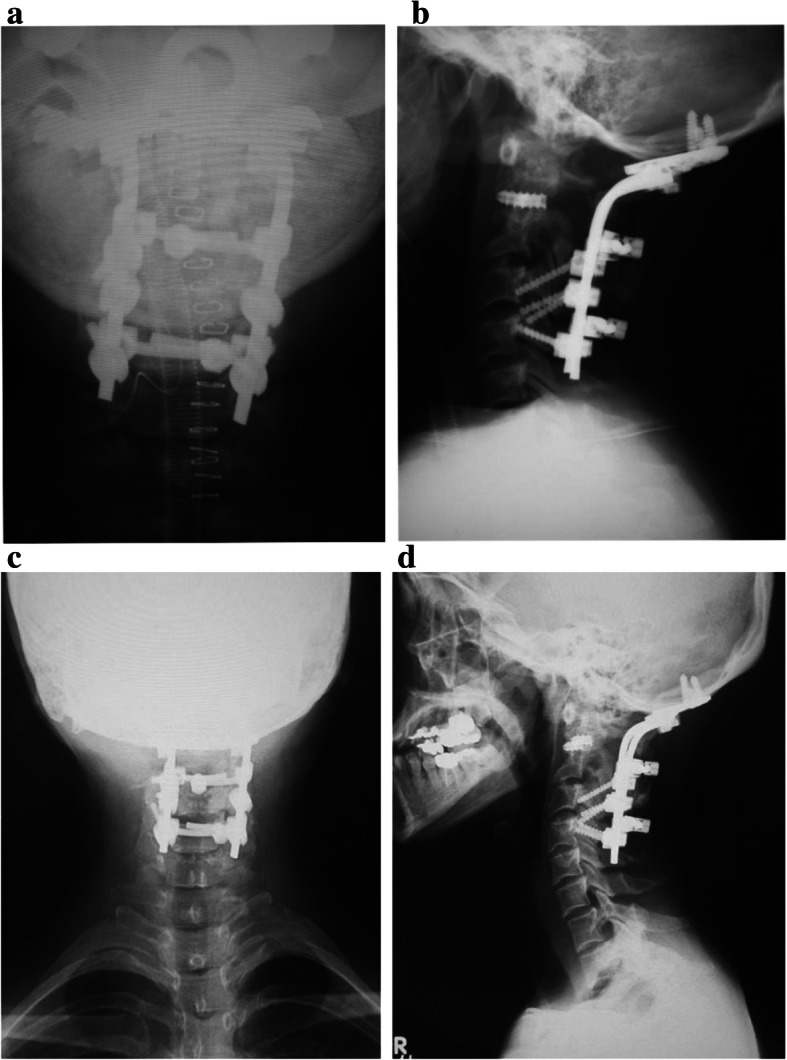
Second operation. a The anterior–posterior radiography image at the second operation. b Sagittal radiography image at the second operation. A long fixation from the occipital bone to C4 was performed. The upper atlantodental interval was 2.8 mm. c Anterior–posterior radiography image at 4 years after the second operation. d Sagittal radiography image at 4 years after the second operation
